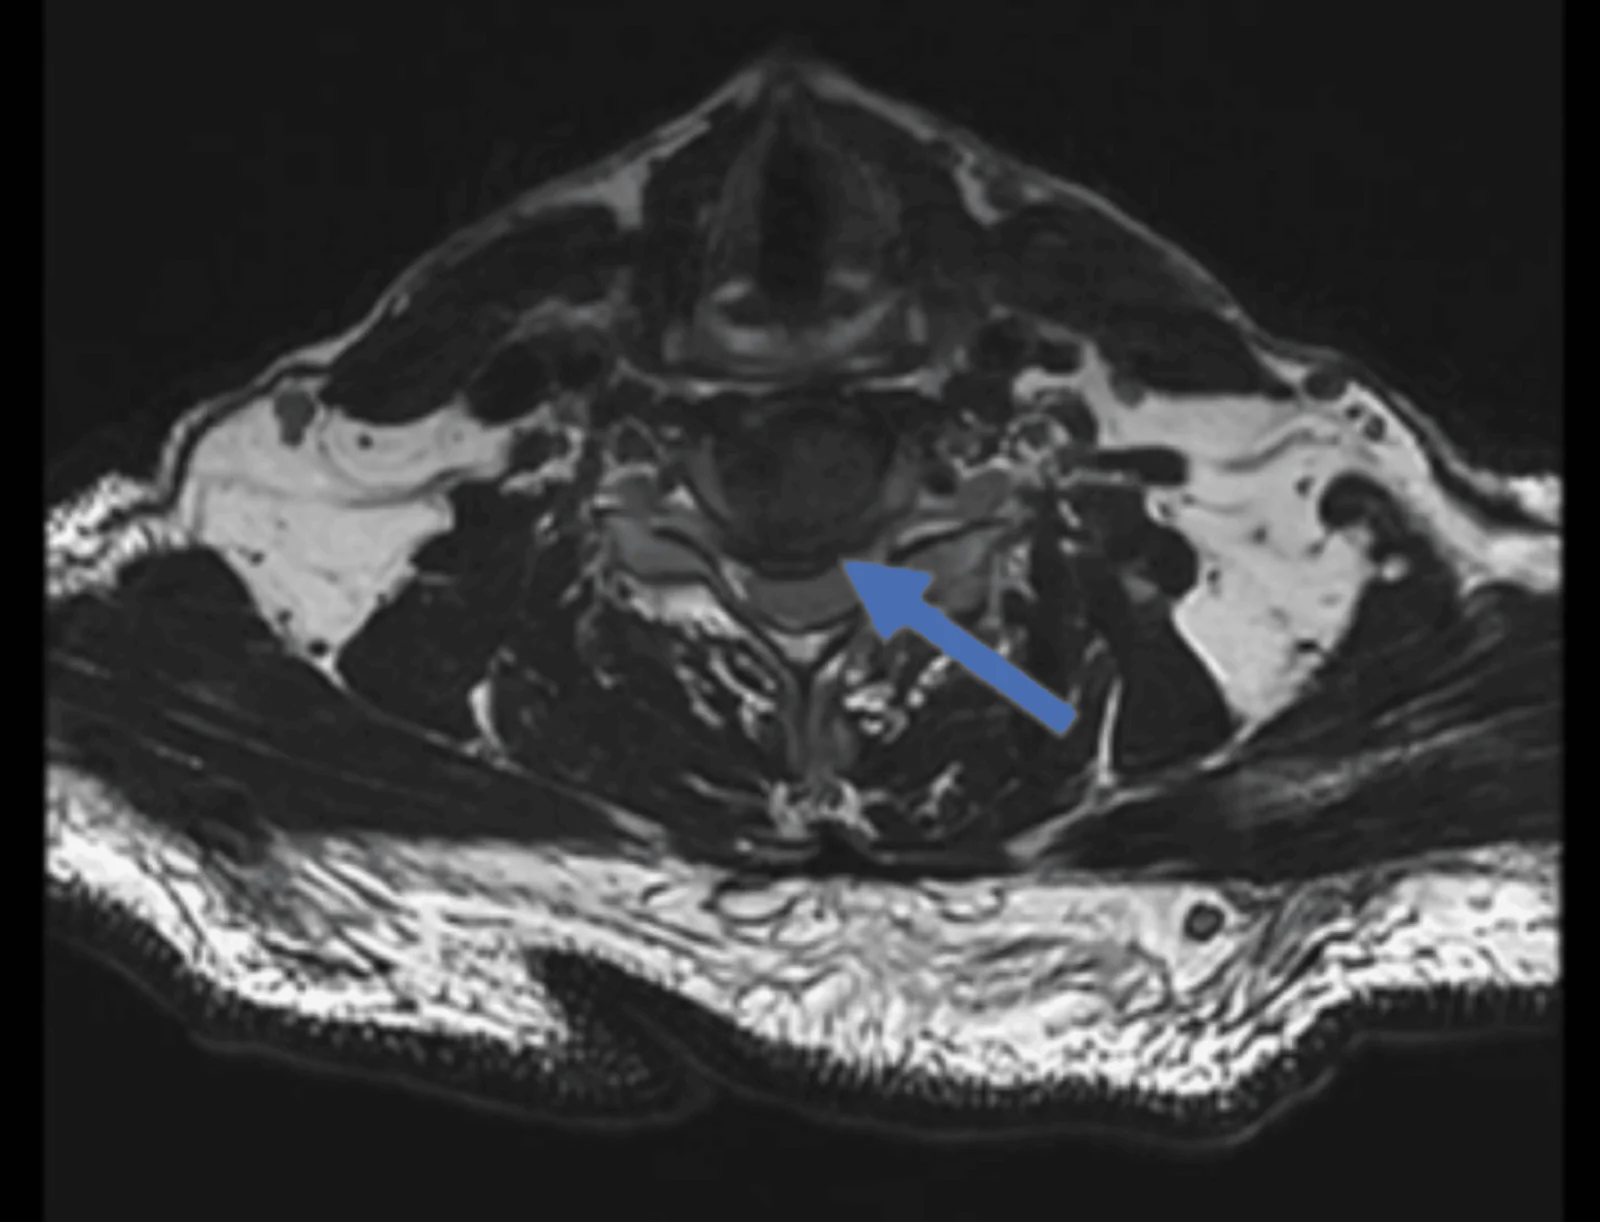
Axial T2-weighted MRI at the C5-C6 level Axial T2-weighted MRI at the C5-C6 level showing significant canal narrowing with marked spinal cord compression (arrow). MRI: magnetic resonance imaging
